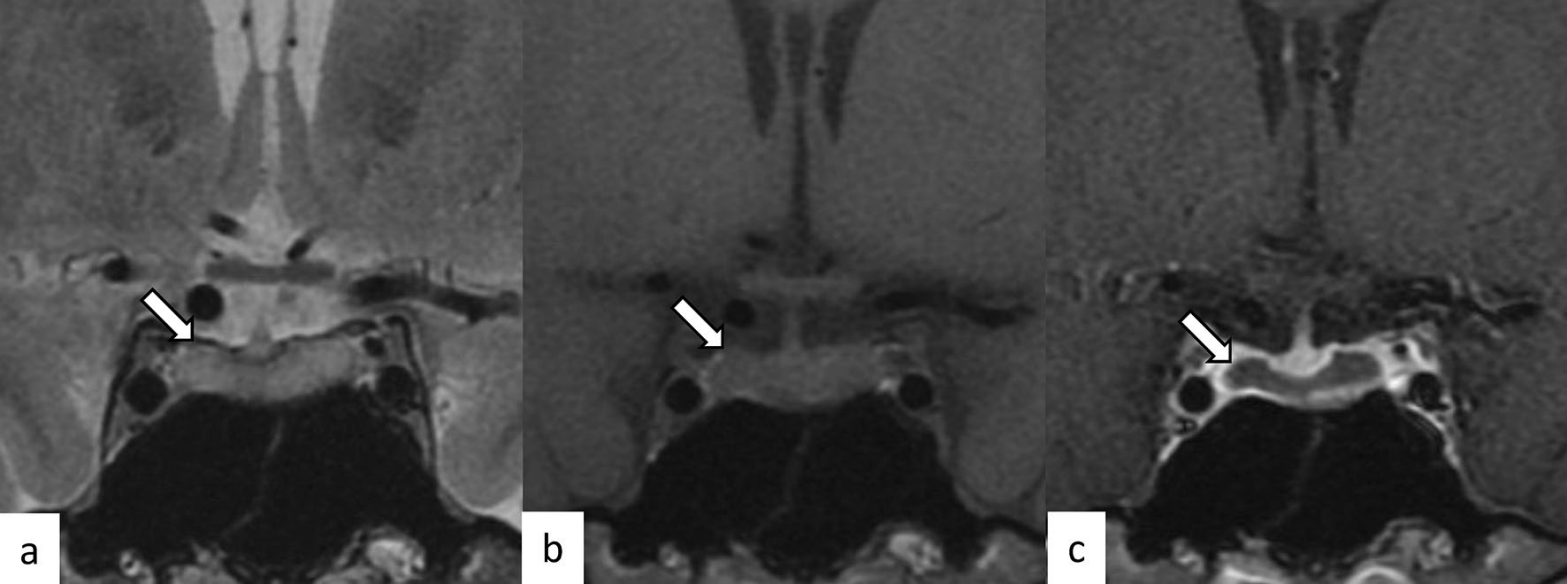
A 33-year-old woman with Sheehan’s syndrome after a cesarean section with massive blood loss. The size of the pituitary gland is within normal limits. The anterior pituitary lobe shows hyperintensity on T2-weighted coronal image (a, arrow), isointensity on T1-weighted coronal image (b, arrow) without contrast enhancement (c, arrow)
